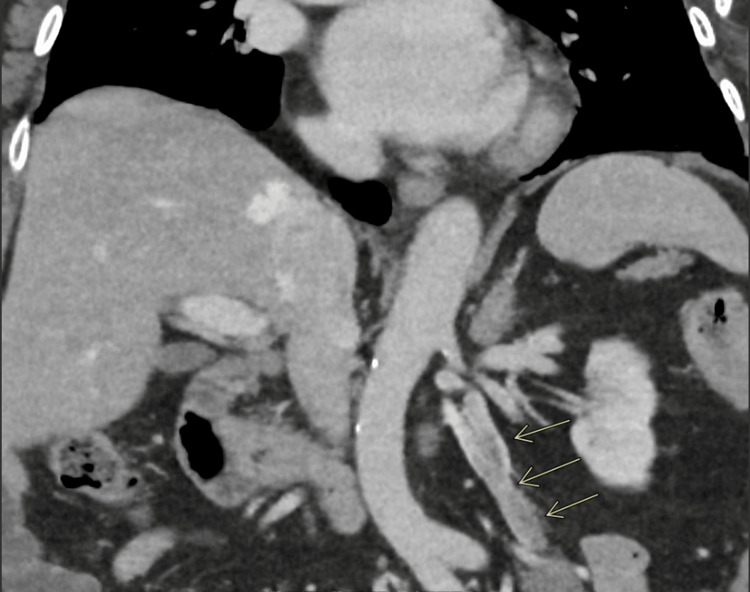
CT scan of abdomen with contrast (coronal view), showing left ovarian vein thrombosis (arrows)
